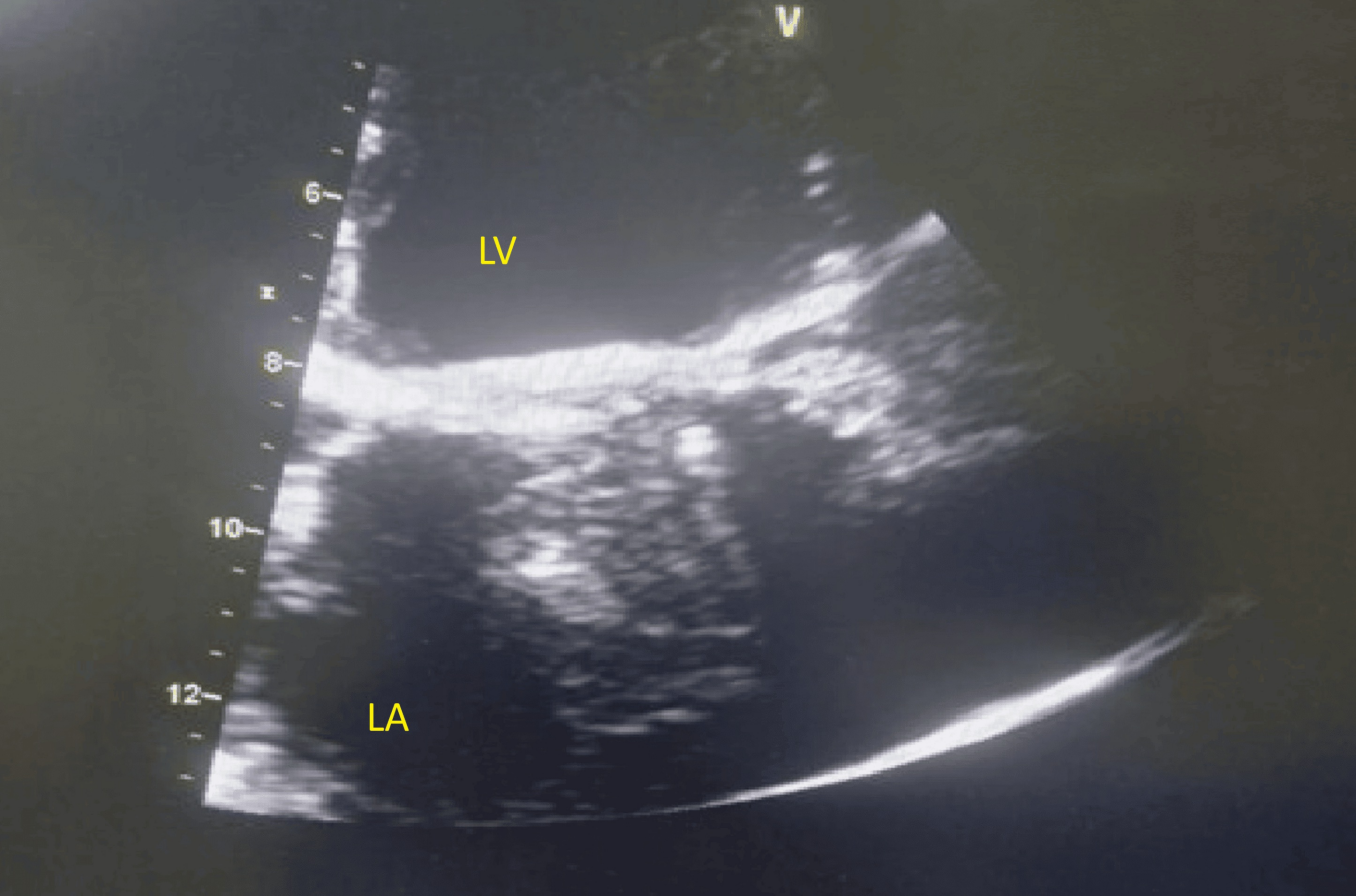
Echocardiography of the second episode Echocardiography of the second episode showing recurrent obstructive thrombus on the mitral prosthesis in a zoomed four-chamber apical view (4C), along with reverberation artifact due to the mechanical mitral prosthesis. LA - left atrium; LV - left ventricle
